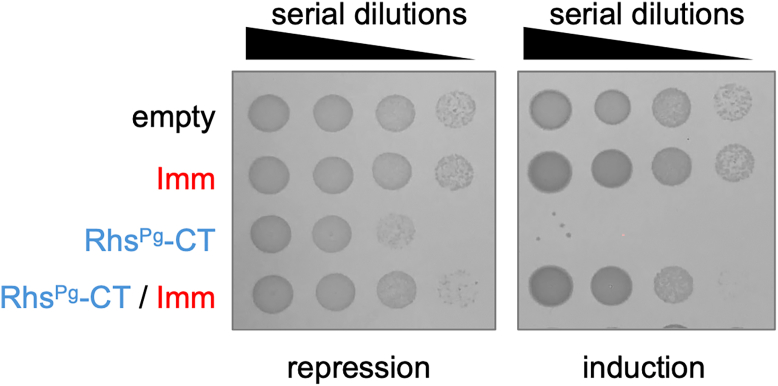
The Rhs^Pg^-CT is toxic in the *E. coli* cytoplasm and is neutralized by the downstream immunity protein. Toxicity assay in the heterologous host *E. coli.* Overnight cultures of *E. coli* cells producing the Rhs^Pg^-CT from the low-copy vector pNDM220, the putative immunity protein (A8H26_06350, Imm) from the pBAD33 vector, or both, were serially diluted (10^−1^ to 10^−4^) and spotted on LB agar plates supplemented with 1% of glucose (*left* panel, repression), or with 0.05 mM of IPTG and 0.2% of L-arabinose (*right* panel, induction). Empty, empty pNDM220 and pBAD33 vectors. LB, Lysogeny Broth; Rhs^Pg^-CT, Rhs^Pg^ C-terminal extension.
